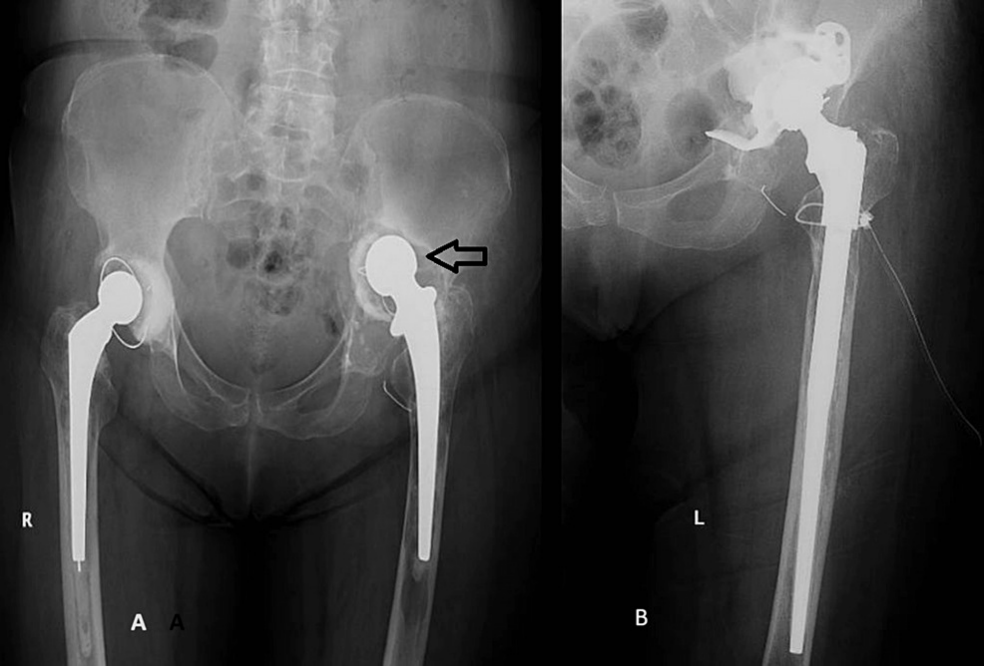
A. Preoperative antero-posterior pelvis X-ray. B. Postoperative left hip X-ray.
